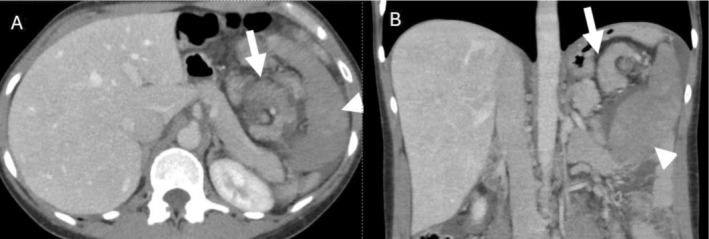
(A) Axial and (B) coronal contrast enhanced CT images showing torsion of the pancreatic tail (arrow) with swirling around the splenic hilum with reduced enhancement consistent with ischemia. The spleen (arrowhead) is enlarged, inferiorly rotated and heterogeneously enhancing due to compromised perfusion.
